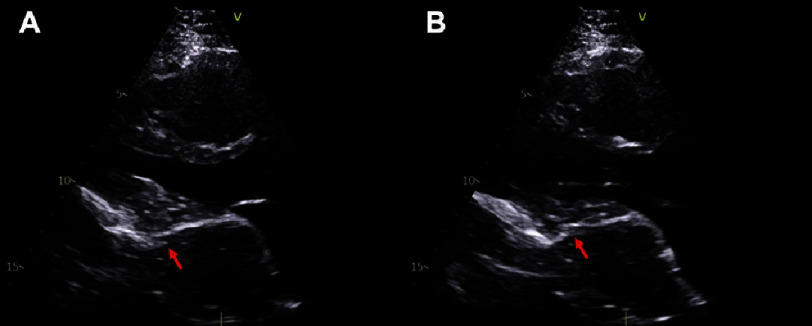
Transthoracic echocardiography of patient with saphenous vein graft aneurysm after symptom onset before first pericardiectomy with exaggerated septal bounce. Systole (A) and diastole (B). Red arrows denote the location of the saphenous vein graft aneurysm.
